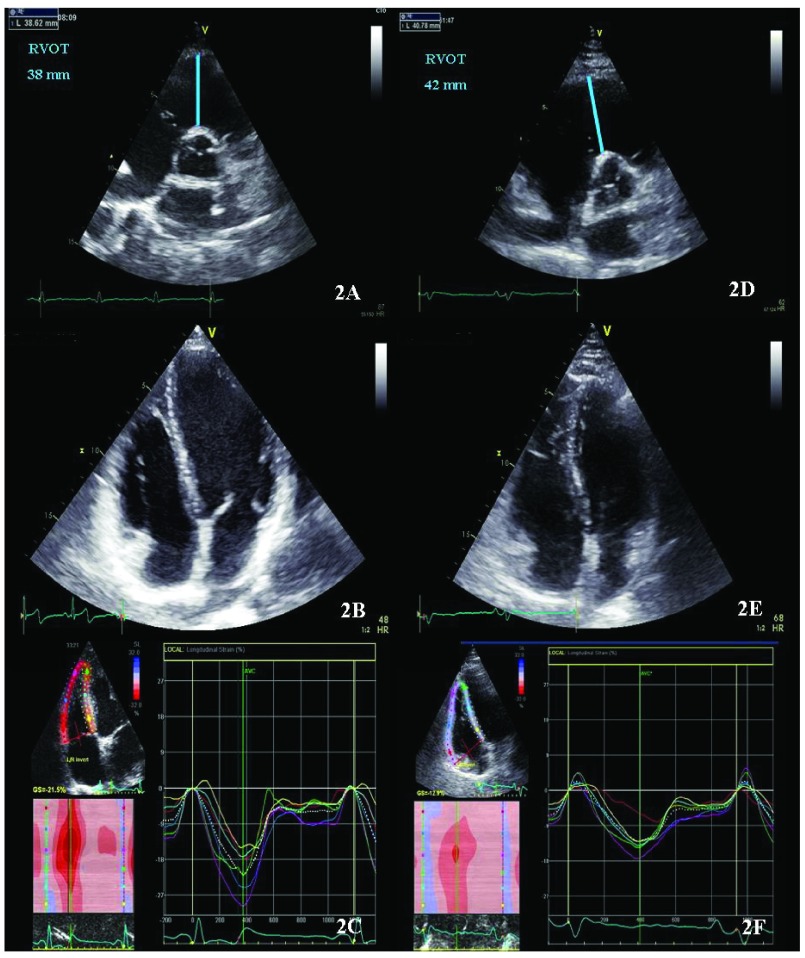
Echocardiographic assessment of an athlete with right ventricle remodelling (Figure 2: A, B, C); and a patient with arrhythmogenic right ventricular dysplasia in early stage (Figure 2: D, E, F). Patients with arrhythmogenic right ventricular dysplasia in early stage can present only mild RV dilatation but the relationship between LV and RV cavity tend to be less than 1 and the RVOT is at least mildly dilated and they present reduced RV global and segmental strain values as compared to athletes.
